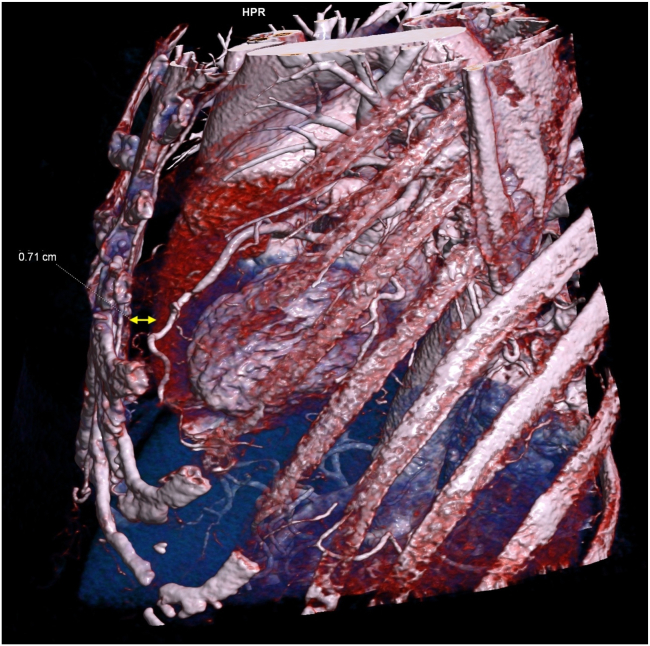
Left lateral view of chest computed tomography with 3-dimensional reconstruction. *Yellow arrow:* Narrowest distance between the sternum and anterior surface of the heart, 0.71 cm.
